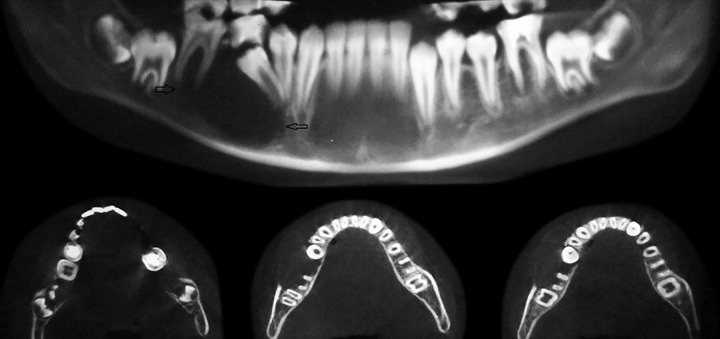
Preoperative CBCT image (Case 5)
